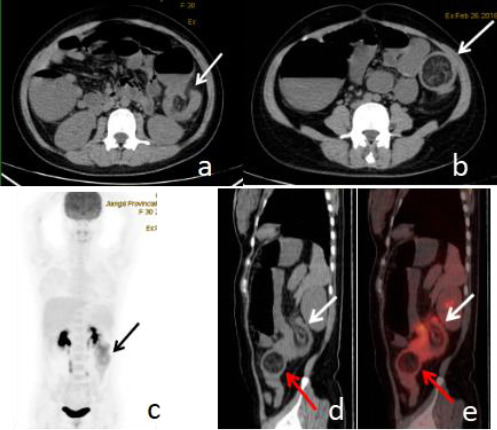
18F-FDG PET/CT of Case 2 showing intussusception due to colonic lipoma. Axial CT showing intussusception (a, arrow) and lipoma (b, arrow). PET MIP image (c) showing hypermetabolic lesion in the left mid-abdomen (arrow). Saggital CT (d) and fusion image (e) showing intussusception (white arrows) and lipoma (red arrows).
